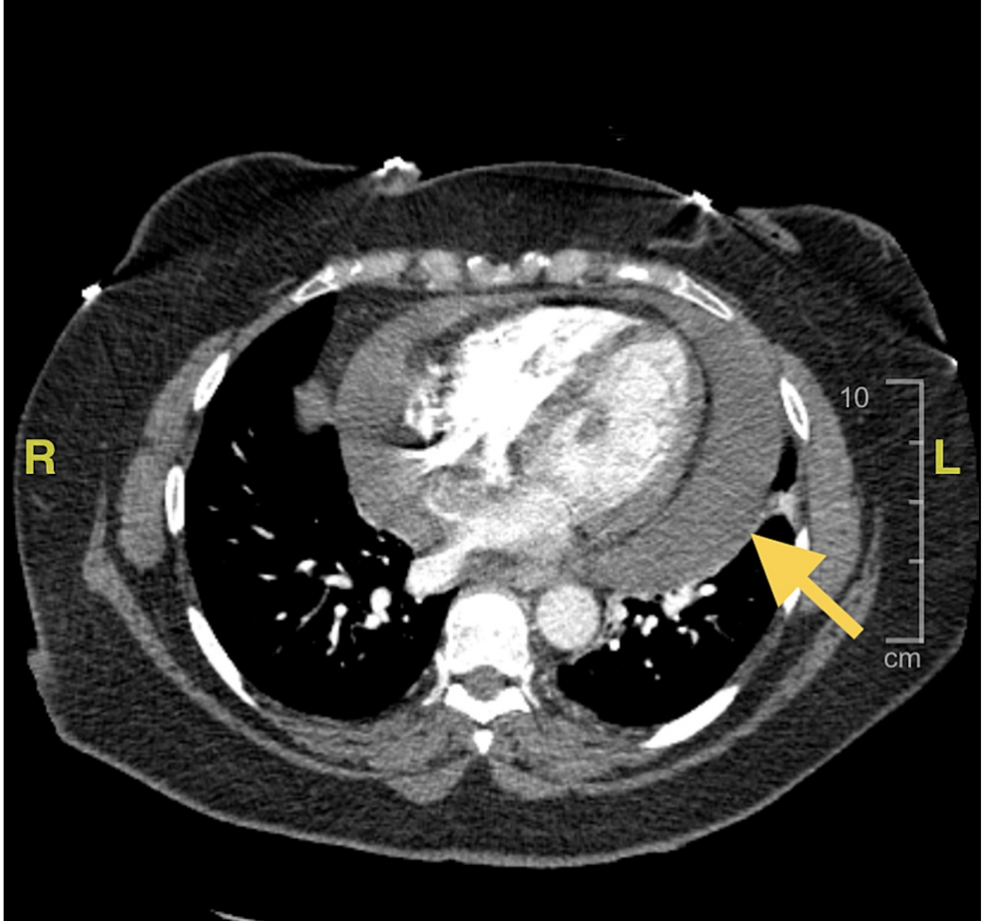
Computed tomography angiography (CTA) of the chest with the yellow arrow showing large circumferential pericardial effusion
